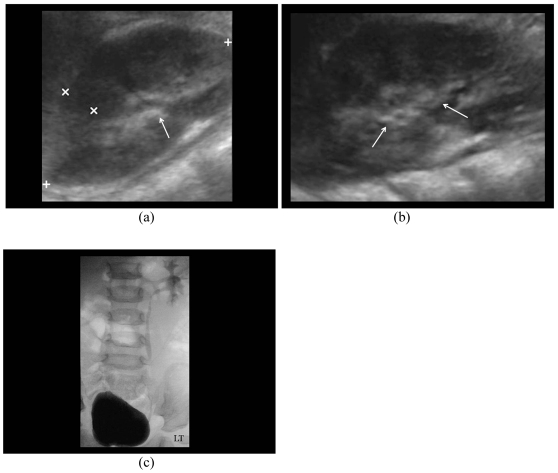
A sixteen months-old girl had one episode of E.coli urinary tract infection. a) Sonogram shows compact sinus echoes (arrow); b) ECS shows air microbubbles in left PCS (arrows) which is not dilated; c) MCU demonstrates left Grade II VUR.
